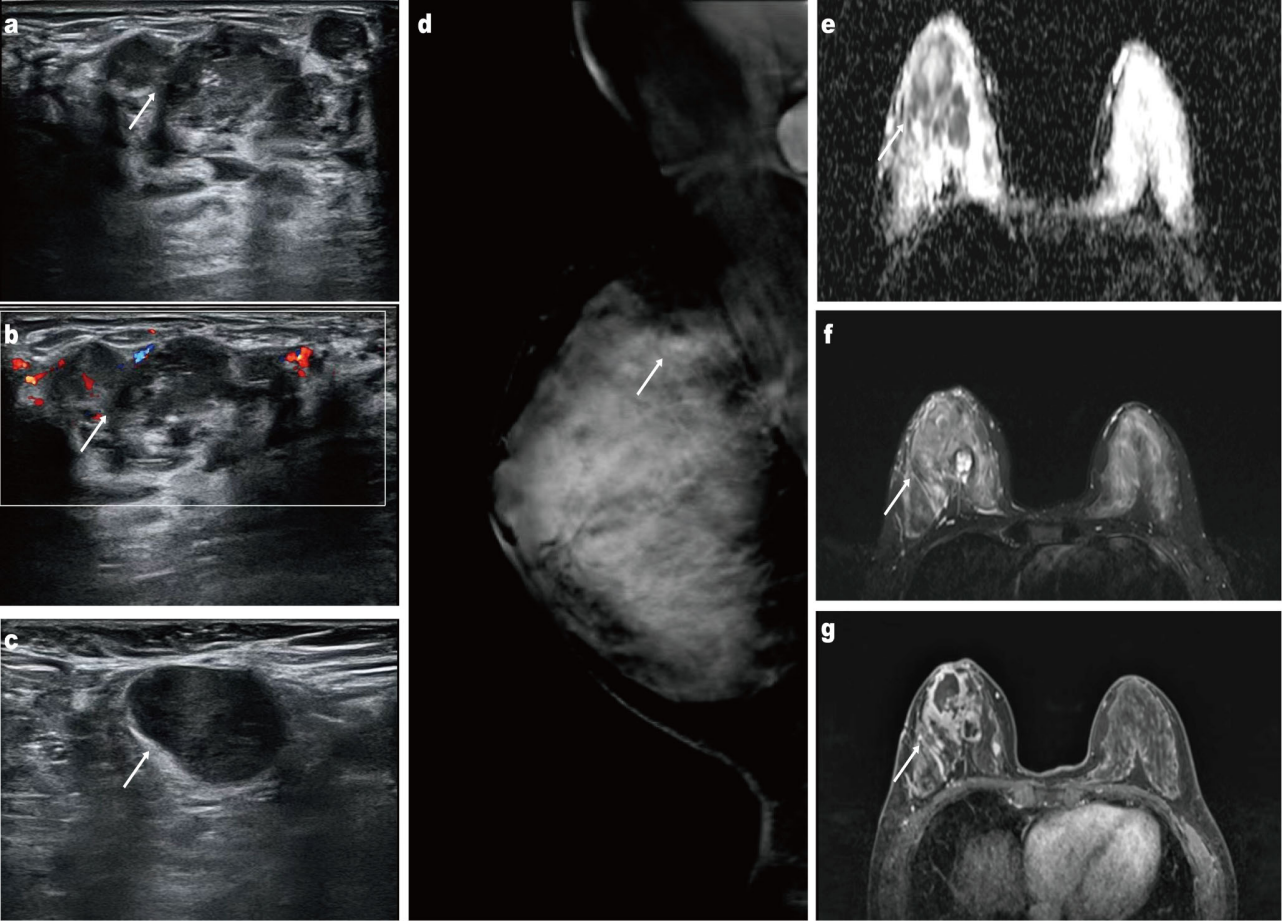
Ultrasonography of the right breast reveals an irregular hypoechoic mass with indistinct margins (a). Color Doppler ultrasonography shows internal vascular flow within the lesion (b). Ultrasonography of the right axilla demonstrates an enlarged lymph node with cortical thickening (c). Maximum-intensity-projection breast MRI image displays a lobulated enhancing mass in the right breast (d). Apparent diffusion coefficient (ADC) map shows markedly restricted diffusion in the lesion (e). Axial contrast-enhanced T1-weighted MRI demonstrates heterogeneous enhancement of the mass (f). Axial T2-weighted MRI reveals high-signal intensity within the lesion (g).
